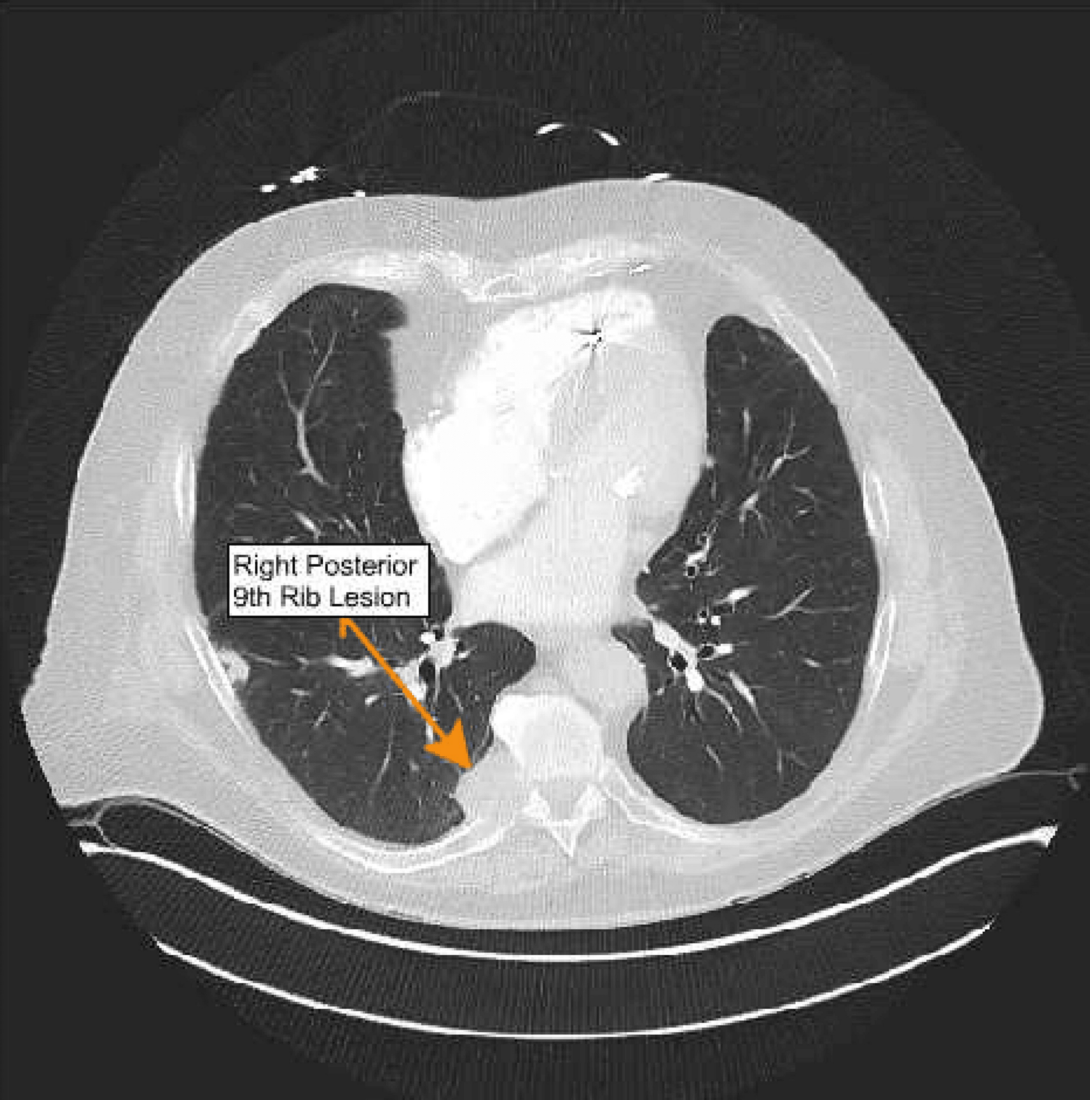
CT image showing right-sided posterior ninth rib lesion
